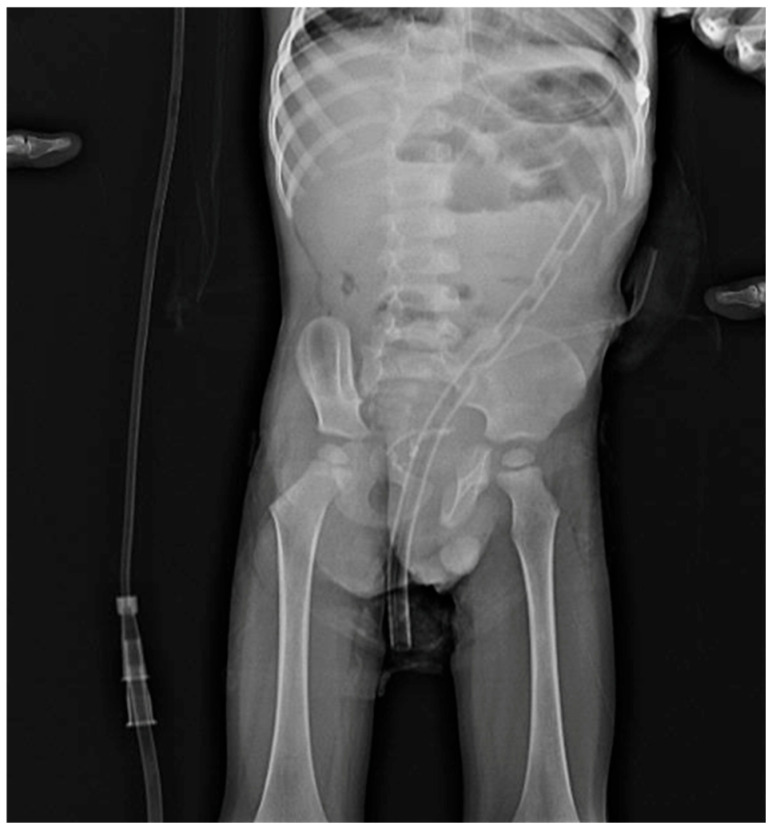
Abdominal X-ray showing the rectal tube and nasogastric catheter in a patient with Hirschsprung-associated enterocolitis (HAEC).
